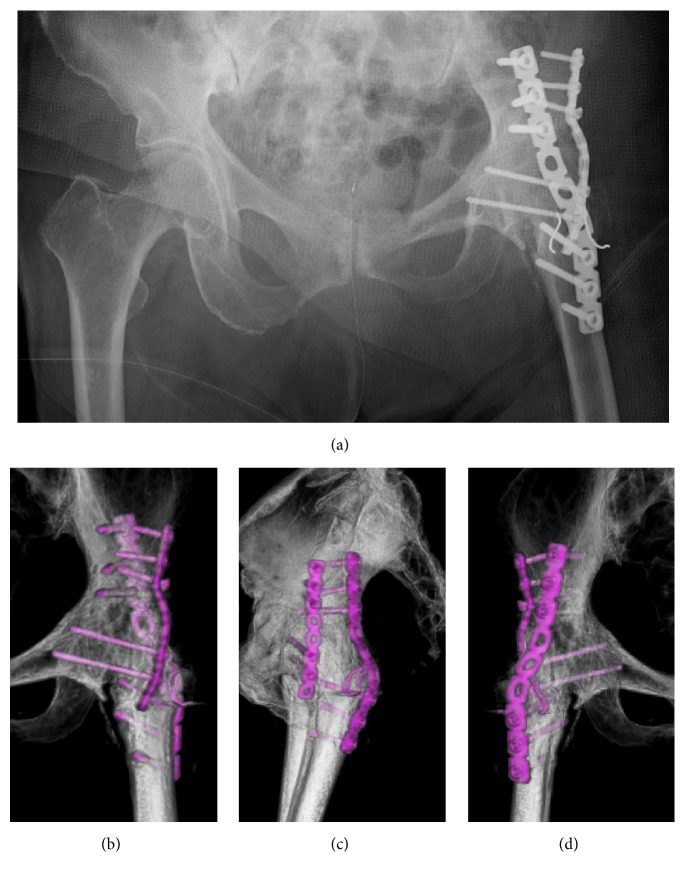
Postoperative images of the hip radiograph and 3D-CT of hip. (a) Radiograph of AP view. (b–d) 3D-CT: (b) AP, (c) lateral, and (d) PA views of the hip. Double-plate fixation positioned at the anteromedial and posterior aspects of the fracture site.
